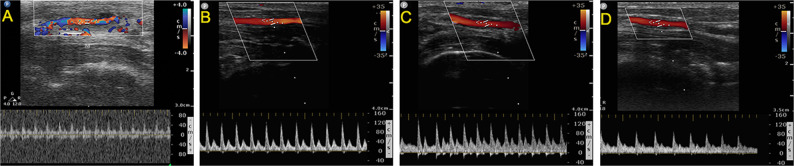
Vascular ultrasound inspection for a rabbit kept patent after implantation at 1 (A), 4 (B), 8 (C), and 12 weeks (D) in the biotube group
